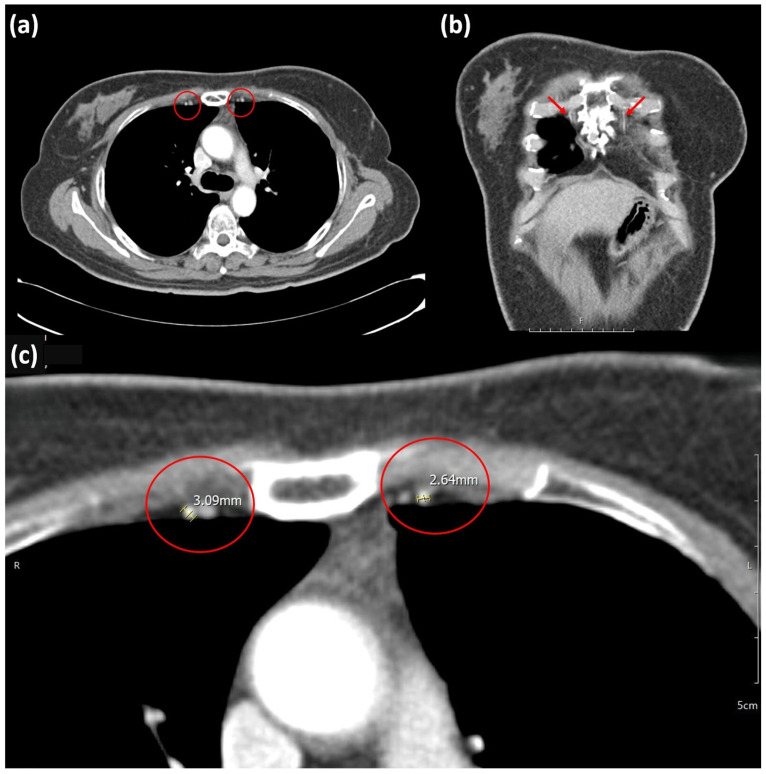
Measurement of the internal mammary artery in CT angiography. (a) Axial CT image with the red circles indicating the anatomical locations of IMAs. (b) Coronal CT image with red arrows highlighting the course of the IMAs. (c) Magnified axial CT image at a magnification of 491.91, with red circles indicating the measurement site of the right and left IMAs. CT, computed tomography; IMA, internal mammary artery.
